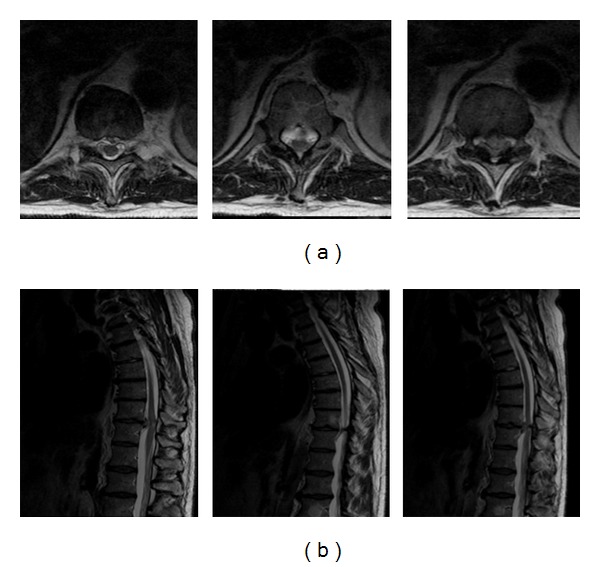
A thoracic spine T2-weighted axial (a) and sagittal (b). MRI showed a central disc herniation at the T10-11 level with compression of the spinal cord with myelomalacia changes at that level. The herniated disc appeared to be soft with no calcified component.
